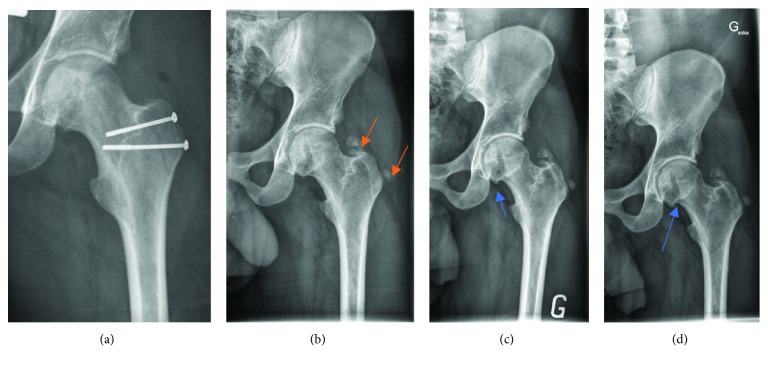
(a) Immediate postoperative AP pelvis radiography showing the two 4.0 mm cannulated screws stabilizing the greater trochanter osteotomy. (b) Two-year postoperative AP X-ray showing good graft position without head collapse or degenerative changes, as well as heterotopic ossification at the tip and lateral to the greater trochanter (orange arrows). (c) Six-year postoperative AP X-ray showing femoral head inferior osteophyte with narrowing of the joint space (blue arrow). (d) Seven-year postoperative AP X-ray showing discrete progression of femoral head inferior osteophyte (blue arrow).
